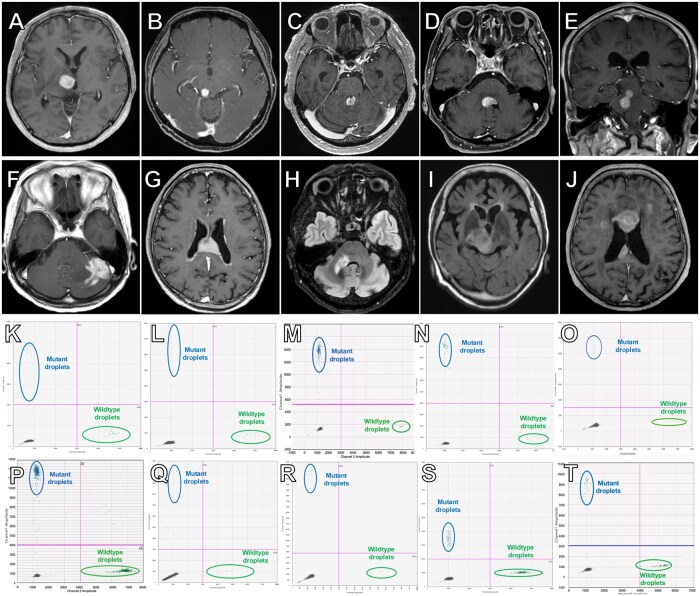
Head MR images of patients #1-10 are shown in (A-J), respectively. (A-G) and (J) are all post-contrast T1 images. (H) and (I) are FLAIR images, because post-contrast MR images could not be taken due to chronic renal failure. The ddPCR results of CSF from patients #1 to 10 are shown in (K-T), respectively. *MYD88* L265P-mutant droplets were detected in all cases. CSF, cerebrospinal fluid; ddPCR, droplet digital PCR.
